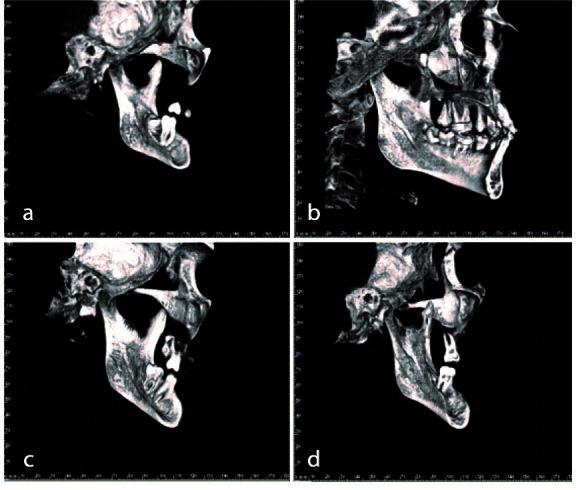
Different types of medial depression of the mandibular ramus (MDMR) based on 3D cone beam computed tomography (CBCT) images, a: circular shape, b: triangular shape, c: tear-drop
